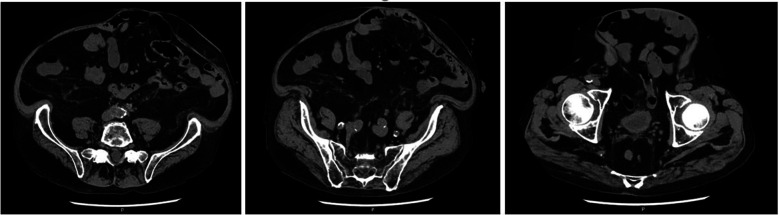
CT scan images at 4 years follow-up of a 71-year-old patient who underwent re-laparotomy 1 month after AAA repair for an ischemic sigmoid perforation and developed a loss of domain hernia due to a severe would infection.
